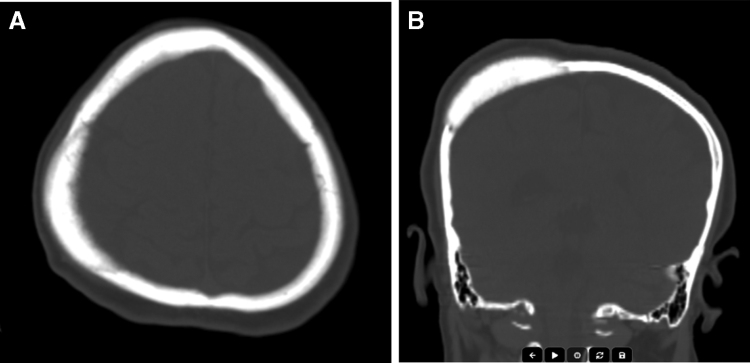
Cranial computed tomography obtained in 2019. (A) Axial bone-window image showing an expansile intraosseous lesion in the right temporoparietal calvarium. (B) Coronal bone-window image demonstrating diploic expansion of the lesion.
